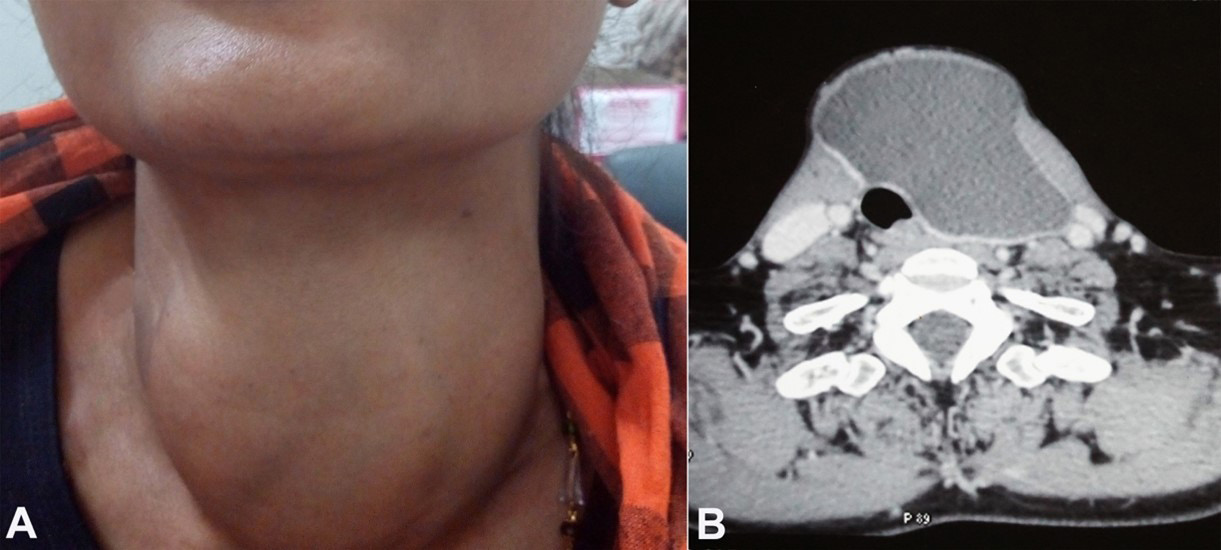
A – Gross view of the diffuse thyroid swelling; B – Contrast enhanced CT scan of the neck showing a large hypodense cystic lesion involving the left lobe of thyroid and partly the isthmus.
